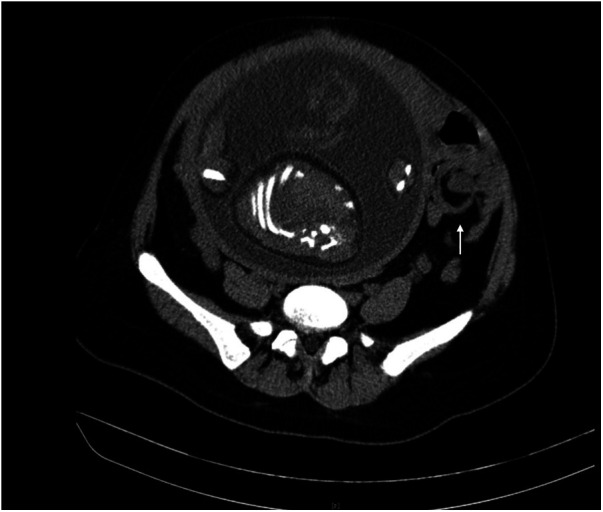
The left lower abdominal part of the small intestine was in a vortex shape in CT.
